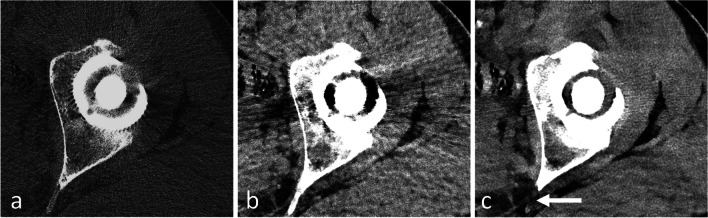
Metal artifact reduction: transverse reformats of PCD-CT of a left total hip replacement acquired at 140 kVp with tin filtration, and reconstructed using different parameters, which affect the metal artifacts and the assessment of the components. a Bone kernel, b virtual monoenergetic image (120 keV) with soft tissue kernel, and c virtual monoenergetic image (120 keV) with soft tissue kernel, and iterative metal artifact reduction algorithm (same window level as in b). Note that the assessment of soft tissue is best performed with the iterative metal artifact reduction algorithm (c), but these algorithms create artifacts mimicking areas of osteolysis (arrow). Bone structures are therefore best assessed without such algorithms (a)
